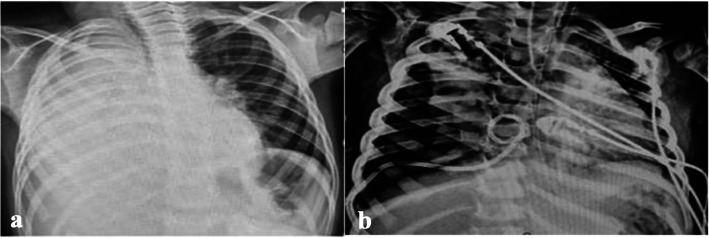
3-year-old male presented with a) severe pneumonia and associated effusion. Lung ultrasound showed highly turbid and septated effusion. b Following administration of intrapleural fibrinolytic, he developed air filled cavities, pneumothorax and worsening respiratory distress requiring escalation of respiratory support to high frequency oscillatory ventilation. Among the study cohort, we reported 3 patients with similar presentation of extensive consolidation and highly septated effusion, and all 3 developed air-filled cavities, BPF and PALS following fibrinolytic administration. This deterioration after fibrinolytics could be part of disease progression to necrotising pneumonia and coincided with fibrinolytics administration, however, we provide alternative hypothesis that administration of fibrinolytics destabilized the fibrinous inflammation which is part of inflammatory reaction in complicated pneumonia. We propose a slower rate of drainage than 10 ml/kg/hour in children, who present with massive effusion and significant underlying lung collapse
